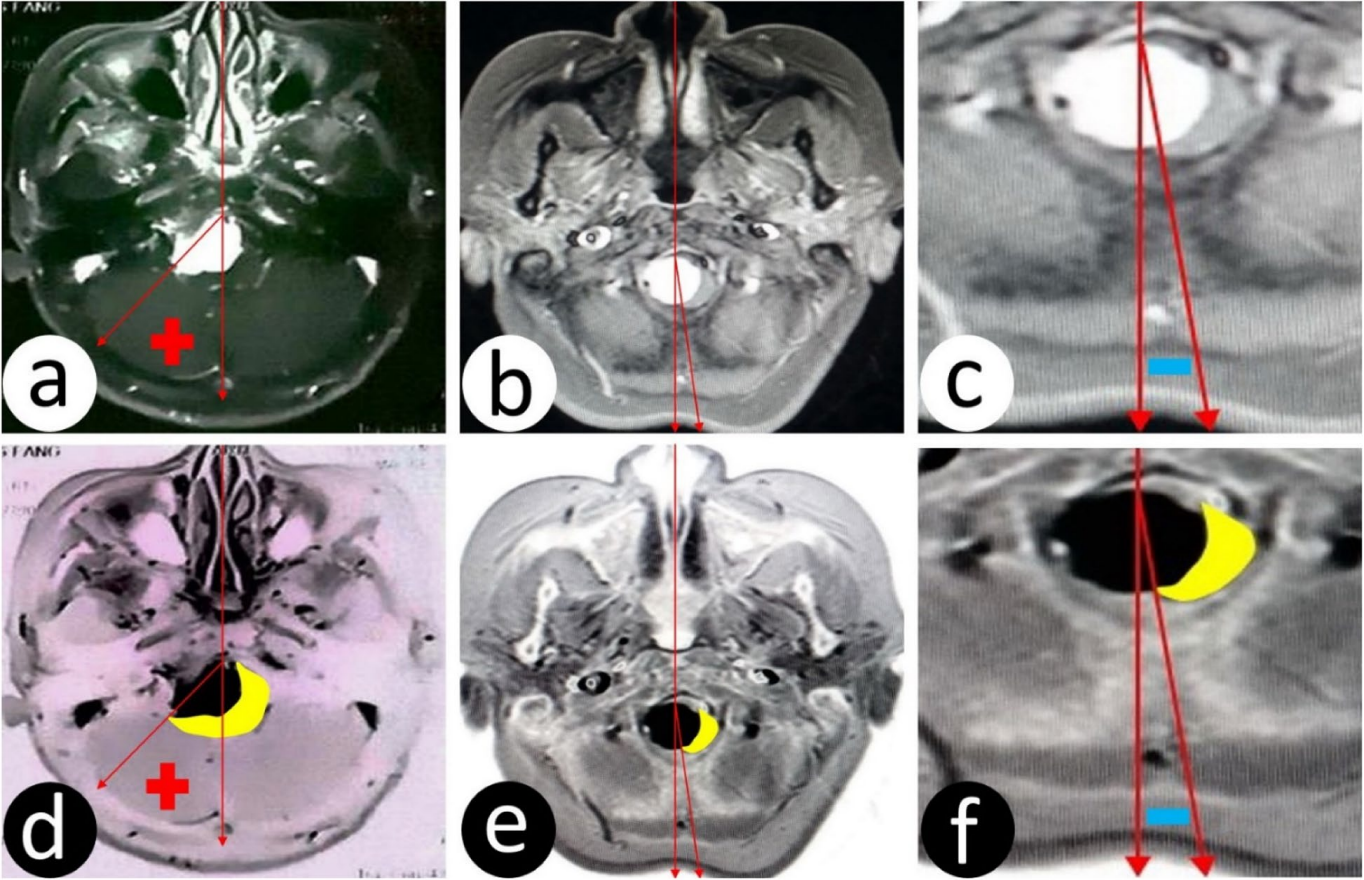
How to define whether a tumor is ahead or on the side of the brainstem? a The maximum level axis tumor enhancement MRI is divided into two equal parts by the median sagittal line, and the pressed brainstem with a lateral displacement is across the median sagittal plane to the contralateral; tumor is believed to be ahead of the brainstem, expressed in the red “+.” b The maximum level axis tumor enhancement MRI is divided into two equal parts by the median sagittal line, and the pressed brainstem with a lateral displacement is not across the median sagittal plane to the contralateral; the tumor is believed to be the side of the brainstem. In the local amplification of b, the tumor is believed to be the side of the brainstem, expressed in the blue “−.” d–f The film in a–c and the yellow part is the brainstem
